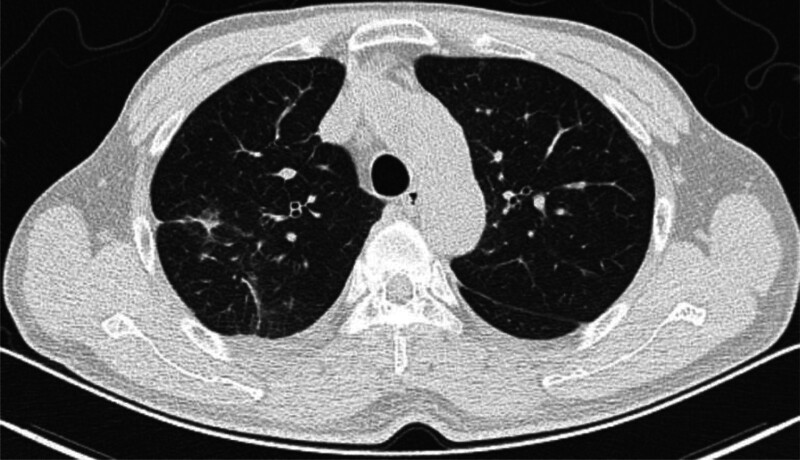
Chest computed tomography scan shows a small amount of inflammation, some fibrous foci in both lungs, and interstitial changes in both lungs with interlobular septal thickening.
